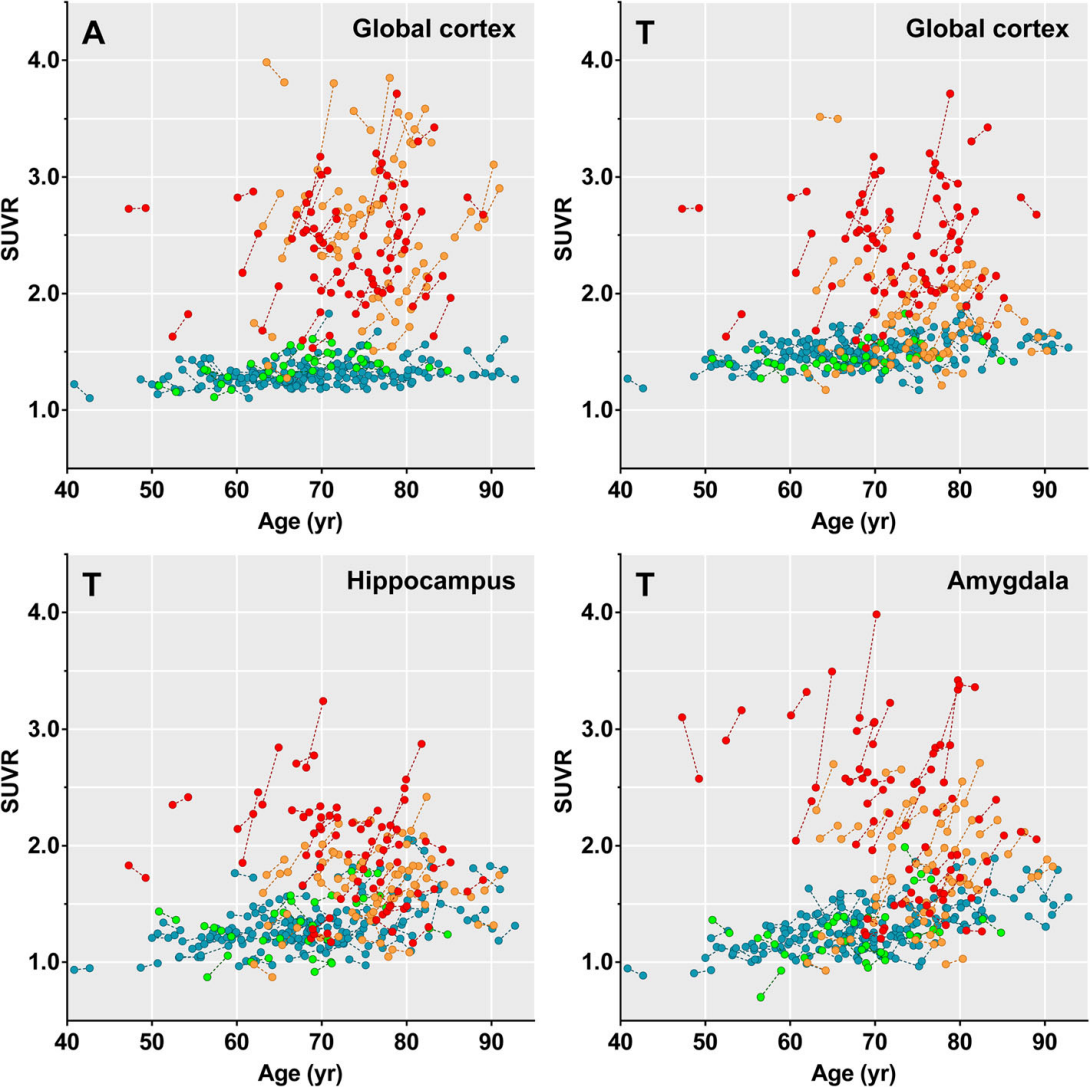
Spaghetti plots showing individual changes in regional SUVR values. In 187 Aβ± individuals, the individual data points measured at baseline and follow-up are displayed as color-coded dots (cyan = Aβ−/ApoE ε4−, green = Aβ−/ApoE ε4+, orange = Aβ+/ApoE ε4−, red = Aβ+/ApoE ε4+). Aβ±, Aβ-positivity; ApoE, apolipoprotein E; SUVR, standardized uptake value ratio; A, ^18^F-florbetaben; T, ^18^F-flortaucipir
